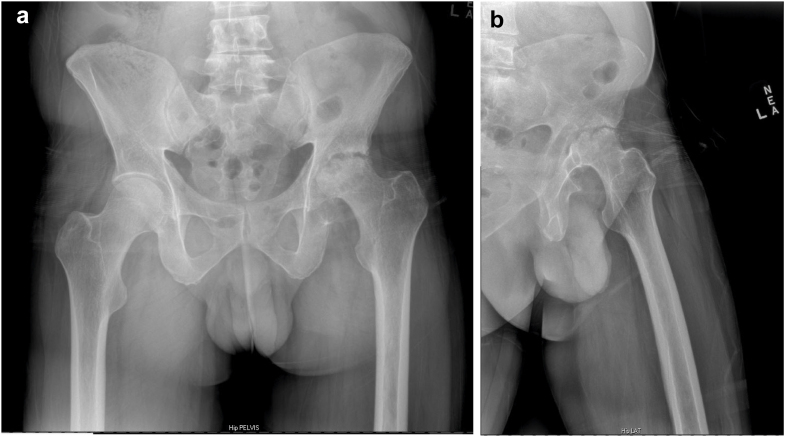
(a) Anteroposterior pelvis X-ray of the left hip showing avascular necrosis of the femoral head. (b) Lateral X-ray of the left hip showing avascular necrosis of the femoral head.
